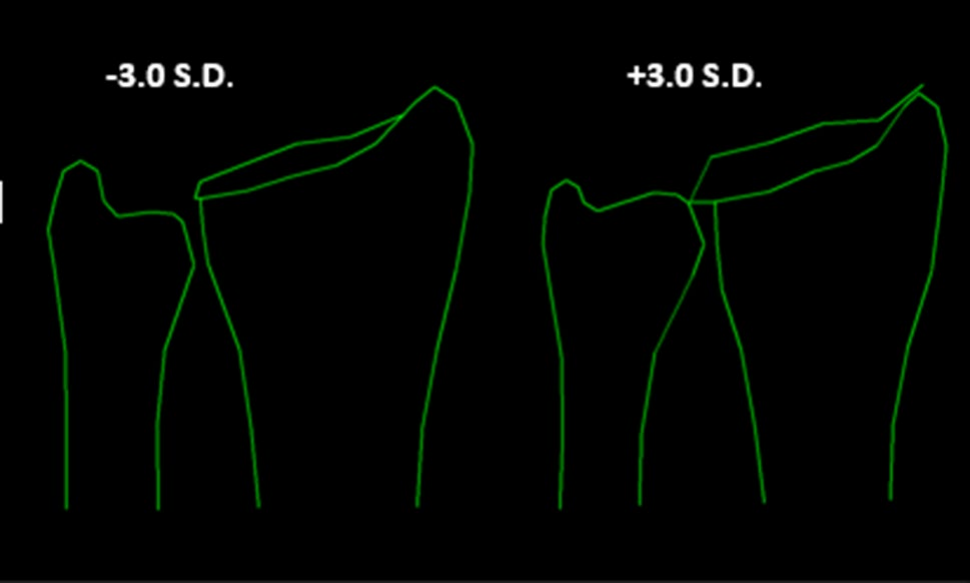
Posteroanterior (PA) mode 4 based on PA radiographs; On the left − 3.0 standard deviation (S.D.) from the mean mode and on the right the 3.0 S.D. from the mean mode. Higher values were associated with a scaphoid fracture
